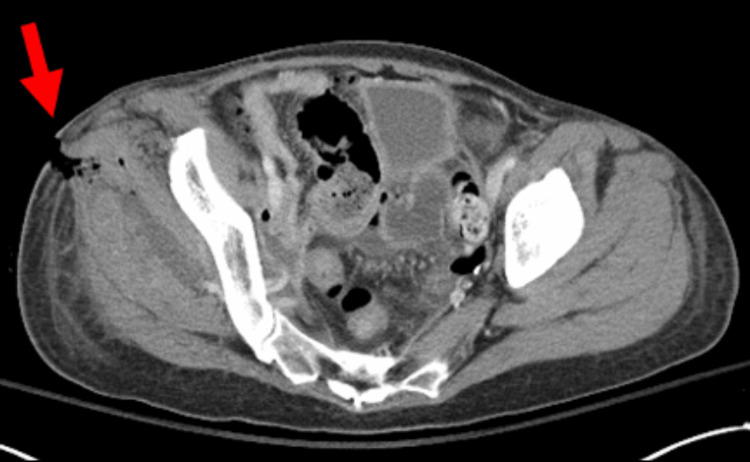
Enterocutaneous fistula. The patient underwent CT imaging of the abdomen and pelvis with oral contrast, which demonstrated an evolving abscess in the right buttocks extending into the pelvis and now in direct communication with the skin (arrow), creating an enterocutaneous fistula.
